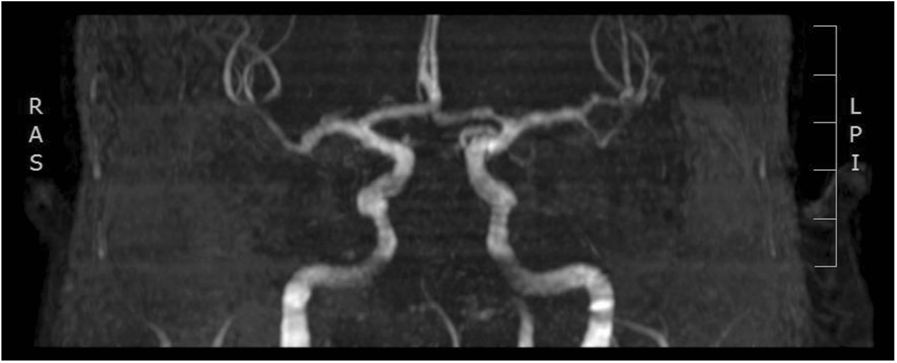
MRI Angiogram showing absence of flow in the basilar artery
